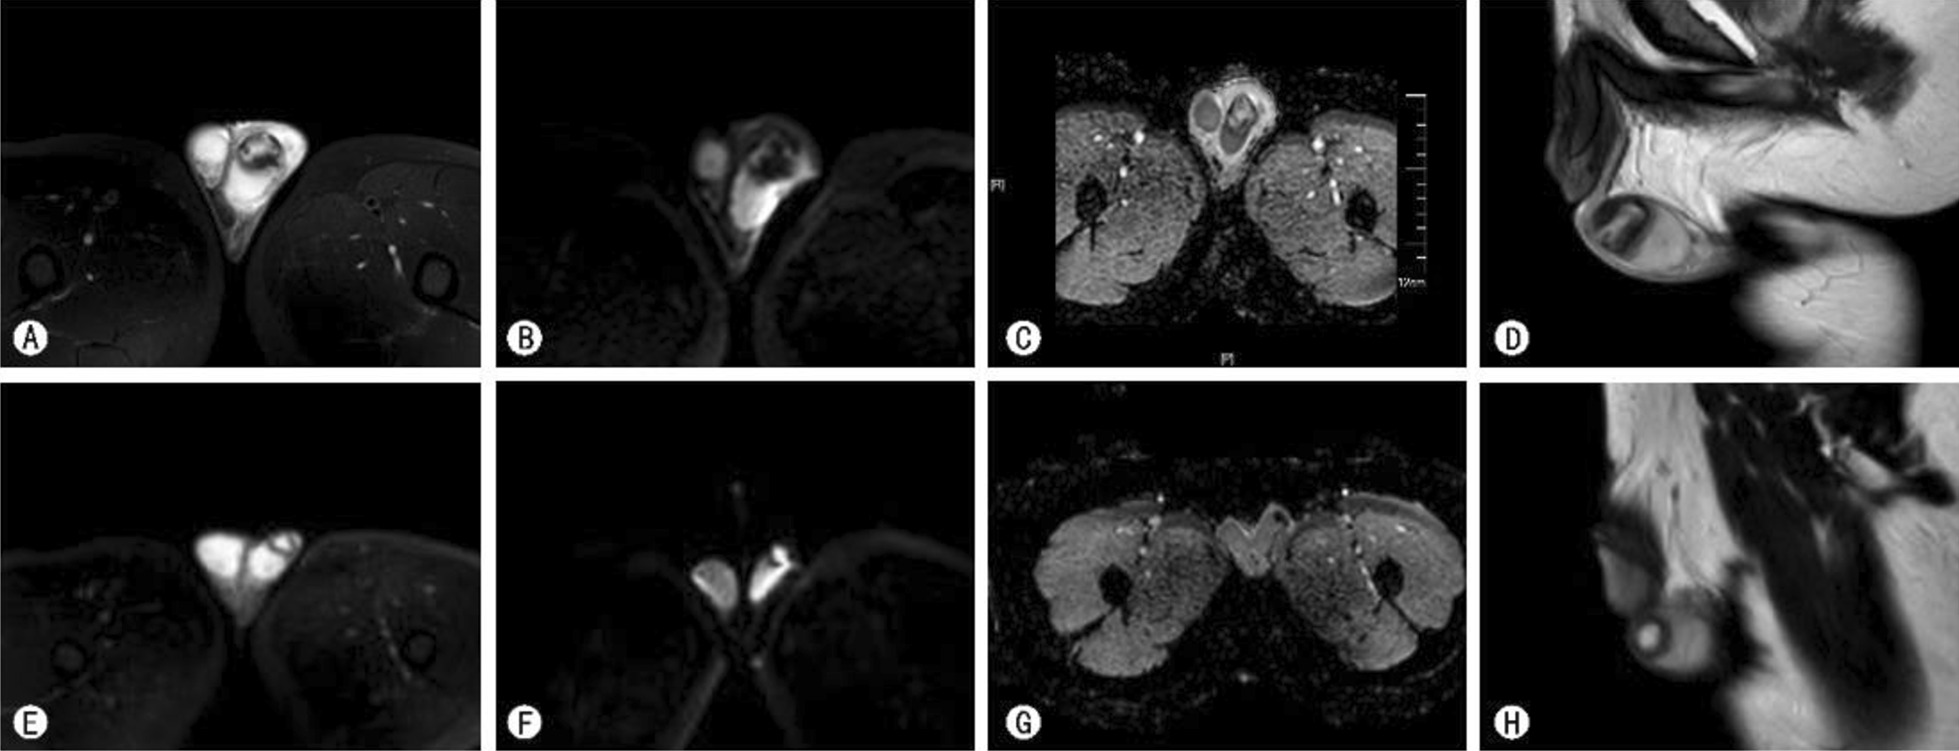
MR examination of the infarction showed a round lesion in the left testis with mixed-signal intensity (low signal intensity on T1WI, mixed high signal and low signal intensity on T2WI, low signal intensity on DWI, mixed high signal, and low signal intensity on ADC). The lesion was with clear boundary, measuring 22 mm * 19 mm, with ring enhancement at the edge, without obvious enhancement (A, B, C, D). Five weeks later, MR revealed a round lesion in the left testis (mixed high signal and low signal intensity on T1WI and T2WI, high signal intensity in the center, low signal intensity at the edge on DWI, and low signal intensity on ADC). The lesion had a clear boundary, measuring 12 mm*12 mm, with ring enhancement at the edge, without obvious enhancement (E, F, G, H)
